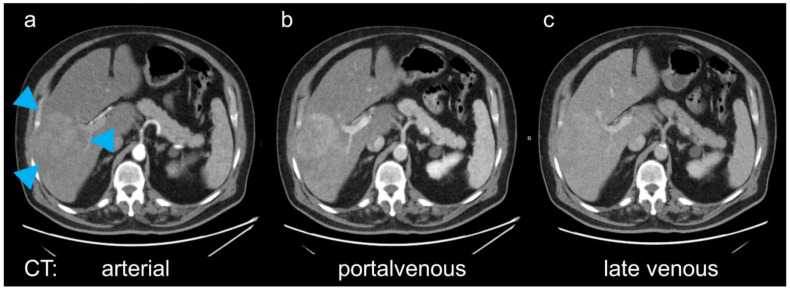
In the presented patient, on computed tomography (CT) the mass in the right liver lobe (a, arrowheads) showed arterial (a) and portalvenous (b) enhancement, with a mixed appearance on the late venous phase with slightly hyper-, but also hypodense areas (c). The diagnostic dilemma was that a biopsy should be avoided, and the patient could not undergo magnetical resonance (MR) imaging because of a pacemaker. Fast dynamic hepatobiliary scintigraphy is limited to planar acquisition, and not feasible for the evaluation of focal lesions [2].
